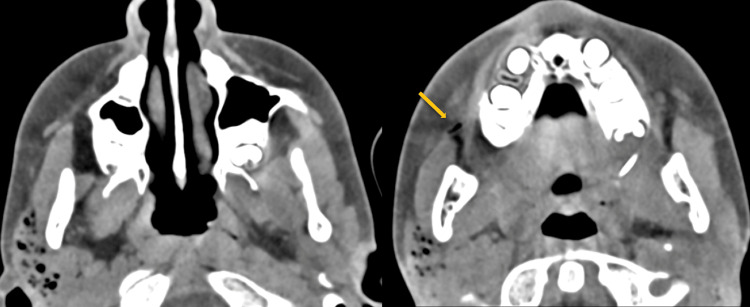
CT scan Axial CT sections demonstrate enlargement of the right parotid gland with multiple millimeter-sized intraglandular gas bubbles and no signs of inflammation. Gas is also observed within Stensen’s duct, extending to the level of the buccinator muscle (arrow).
